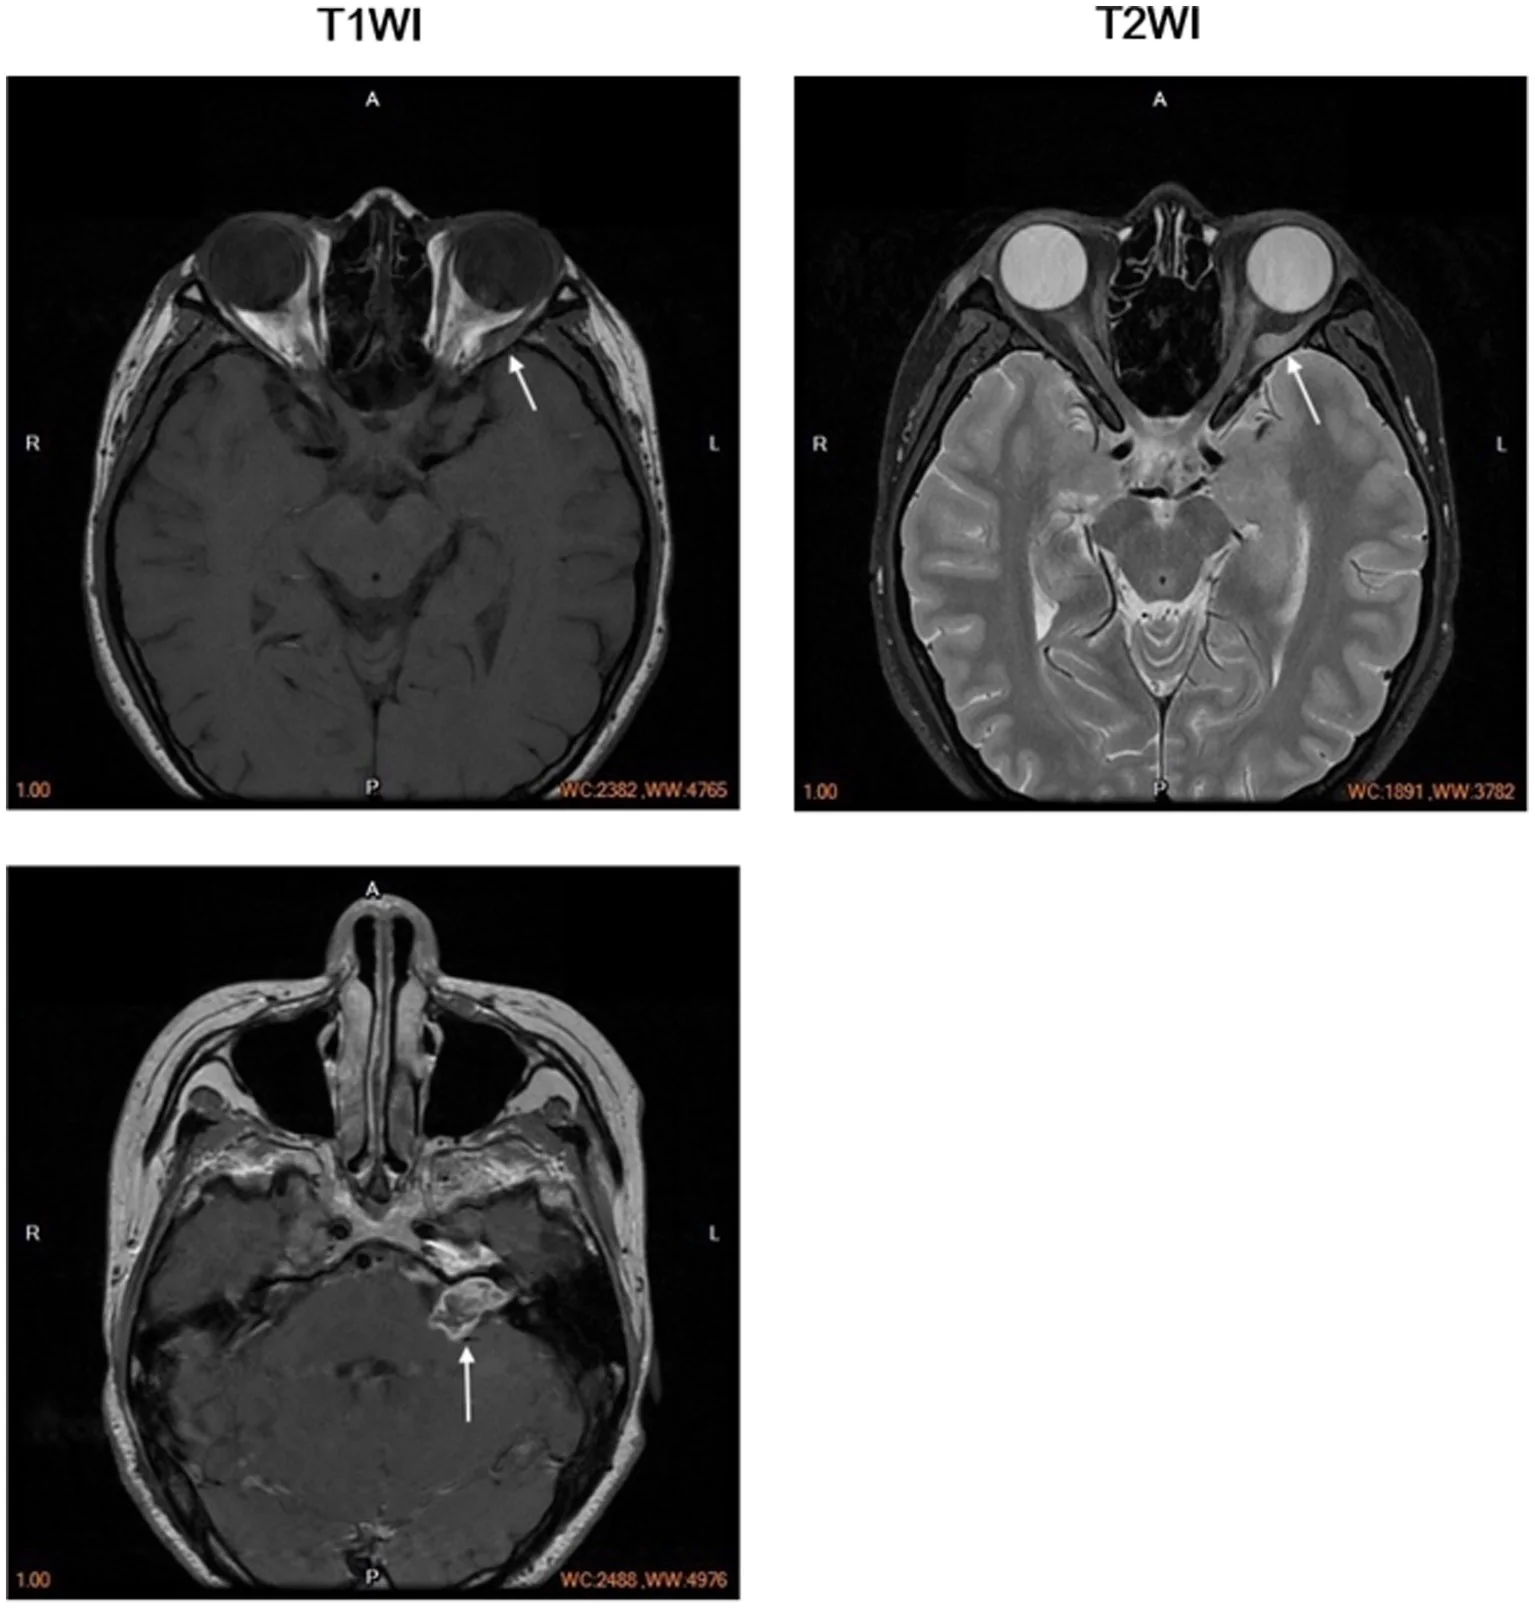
MR images. A nodule that appeared isointense on T1-weighted imaging and hyperintense on T2-weighted imaging was observed superior to the lateral rectus muscle of the left eye. The lesion measured approximately 6 mm x 8 mm, had a clear margin, and showed marked homogeneous enhancement. Additional lesions were observed along the left auditory nerve, bilateral trigeminal nerves, cervical spinal cord, subdural region of the cervical spinal canal, and intracranial meninges, with heterogeneous enhancement and patchy non-enhancing areas.
